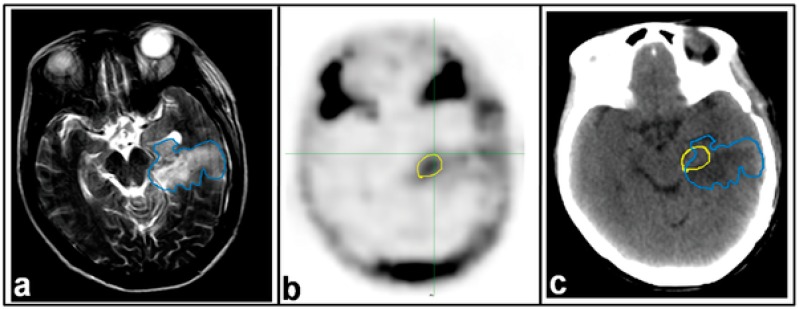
Magnetic Resonance Imaging (MRI) and ^18^F–Fluro-ethyl-tyrosine (^18^F–FLT)/Positron Emission Tomography/Computed Tomography (PET-CT) images from a patient with glioblastoma (GBM). Images were taken 21 days post-operatively and 2 days before radiotherapy. (a) T2-weighted MRI; (b) ^18^F–FLT PET image; (c) CT image of PET-CT scan. Residual tumor regions defined by T2-MRI (blue line) and ^18^F–FLT PET (yellow line) are superimposed on the CT image. This research was originally published in Reference [18].
